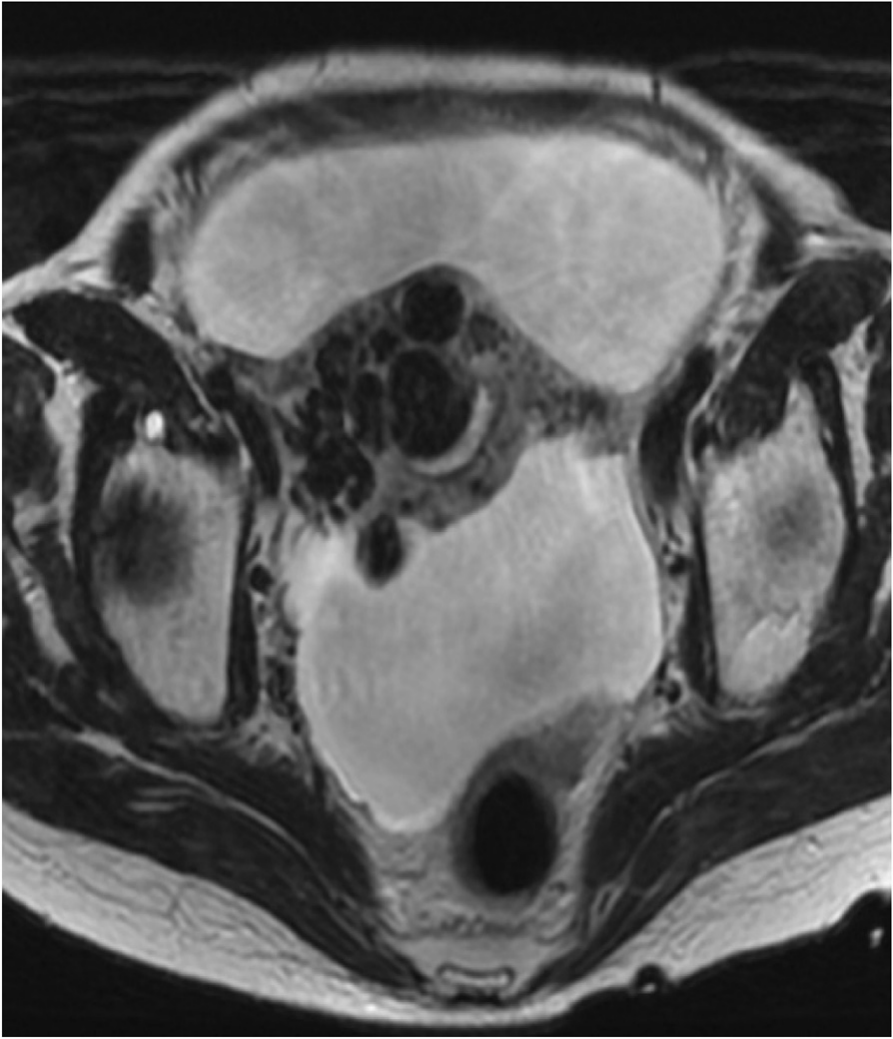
T2-weighted transverse magnetic resonance imaging (MRI) shows multiple tortuous vessels, especially in the anterior uterine wall. Ascites caused by the patient’s idiopathic portal hypertension is also present.
